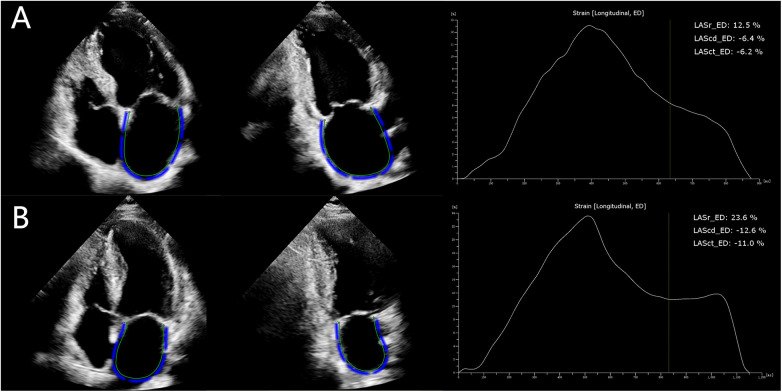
Two-dimensional echocardiographic views of the left atrium (four- and two-chamber views) and three functional phases in severe AS patients with LVEF < 50% (A) and those with LVEF ≥ 50% (B). AS, aortic stenosis; LVEF, left ventricular ejection fraction; LASr, left atrial reservoir strain; LASct, left atrial contractile strain; LAScd, left atrial conduit strain.
